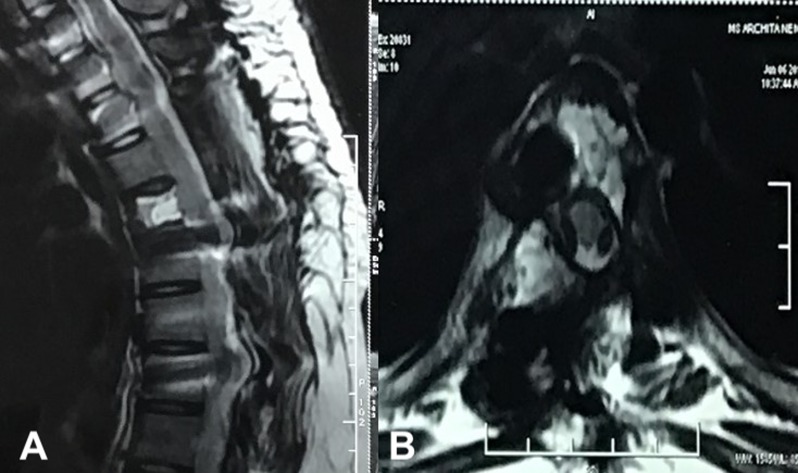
A 6-month follow-up MRI post completion of Denosumab therapy. (A) shows a sagittal T2WI MRI image with resolution of previous dorsal compressive mass and (B) axial image of the decompressed spinal cord.
